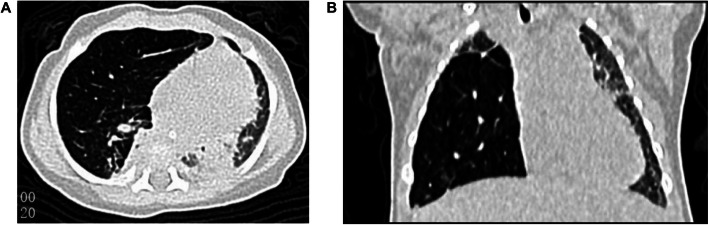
Chest CT at 73 days after birth revealed emphysema in the middle lobe of the right lung with mediastinal hernia
